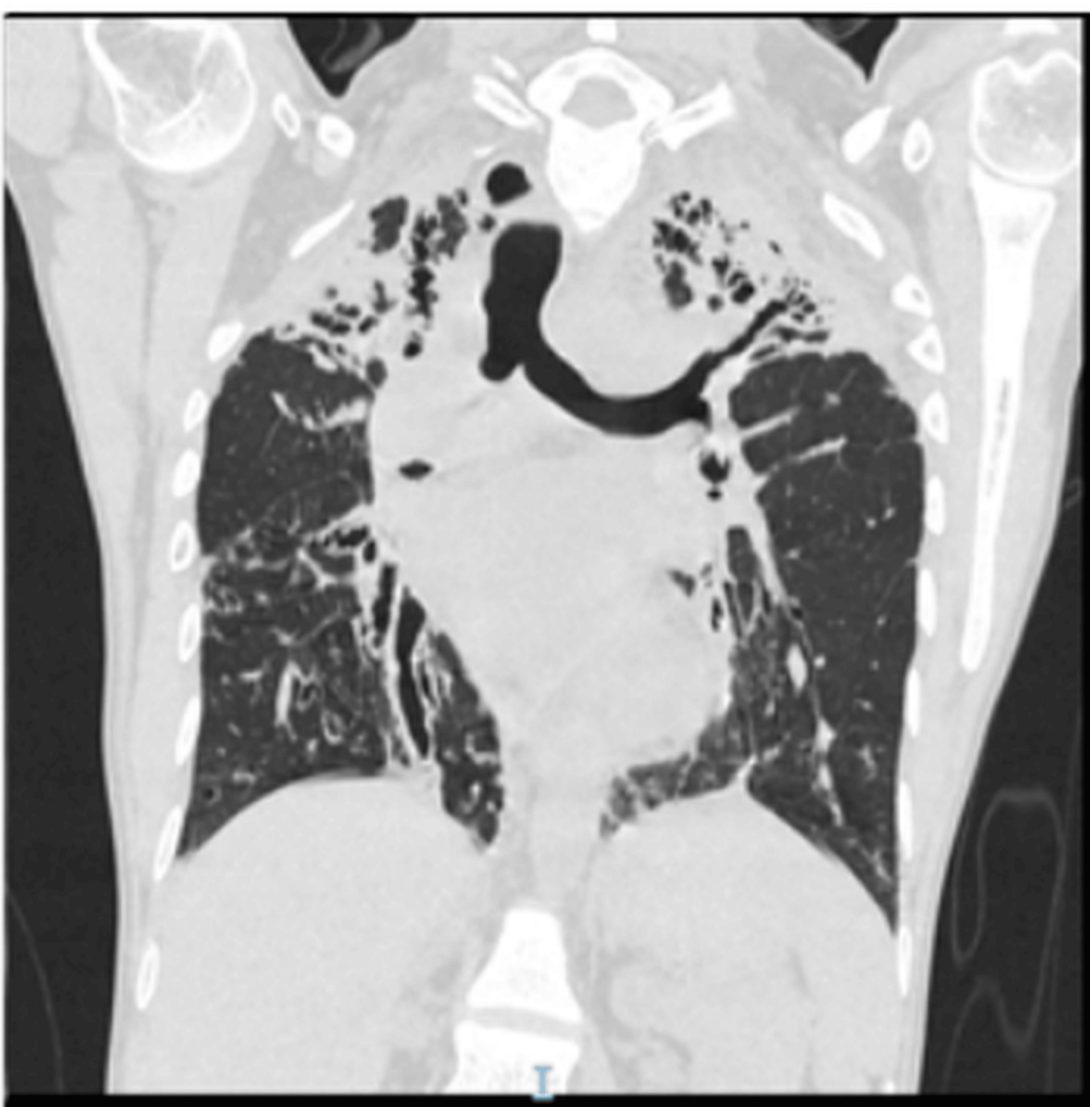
High-resolution CT of the chest revealing interval progression The follow-up high-resolution CT of the chest shows marked progression of bilateral cylindrical bronchiectasis with worsening mucous plugging and increased distribution of infiltrates. Varicose bronchiectasis was noted in the right lung, particularly in the lower lobes, indicating architectural distortion and chronicity. Compared to Figure 1, this scan shows an interval worsening with an increased extent of bronchiectasis and more prominent parenchymal changes, including denser infiltrates and volume loss, most notably on the right side.
